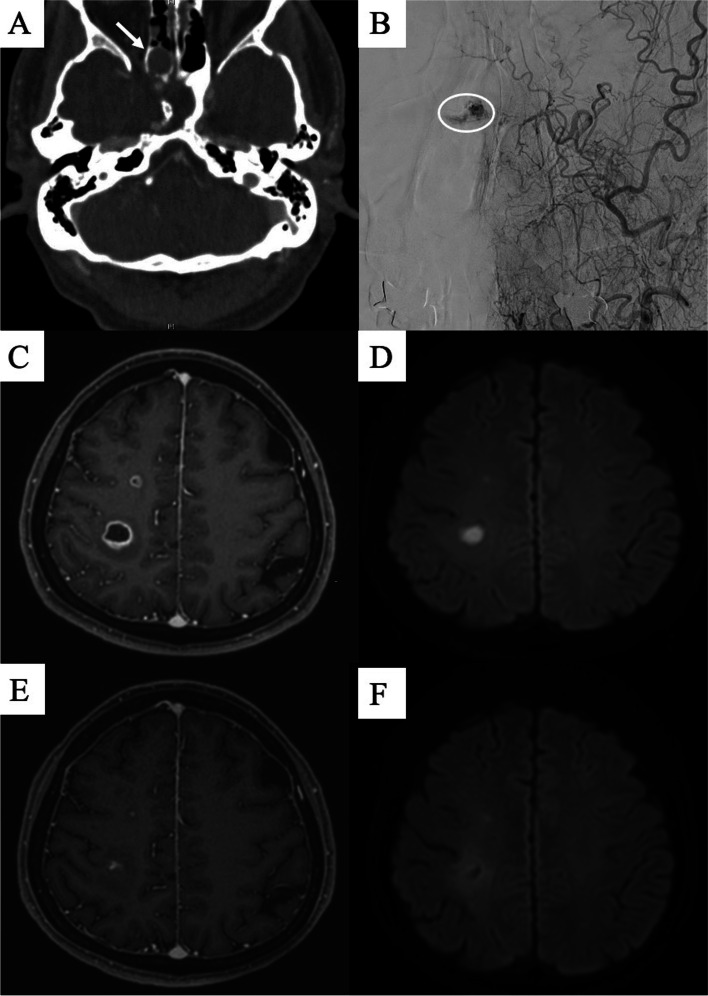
Evidence of chronic inflammation in the right nasal cavity. a Granulation ball with slight enhancement on brain CT, *indicated by white arrow*. b Left external carotid artery angiography shows a small granulated mass with capillary filling, *indicated by white circle*. Multiple brain abscesses in the right hemisphere show c peripheral enhancement on a brain MRI T1-enhanced image and d diffusion restriction on a diffusion-weighted image. Brain MRI at the 1-month follow-up visit shows regression of the abscesses on e T1-enhanced image and f diffusion-weighted image
